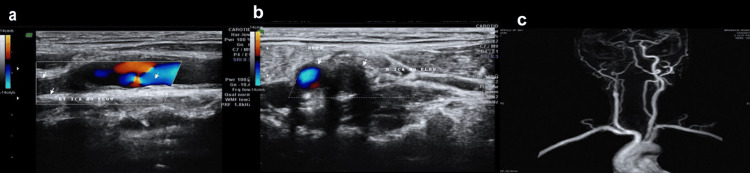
Imaging findings of right internal carotid artery occlusion a: color Doppler ultrasonography shows no flow in the right internal carotid artery (ICA) (arrows); b: longitudinal Doppler view confirming the occluded segment of the right ICA with no detectable flow (arrows); c: magnetic resonance angiography (MRA) demonstrating complete occlusion of the right ICA with preserved flow in the contralateral carotid and vertebrobasilar circulation.
